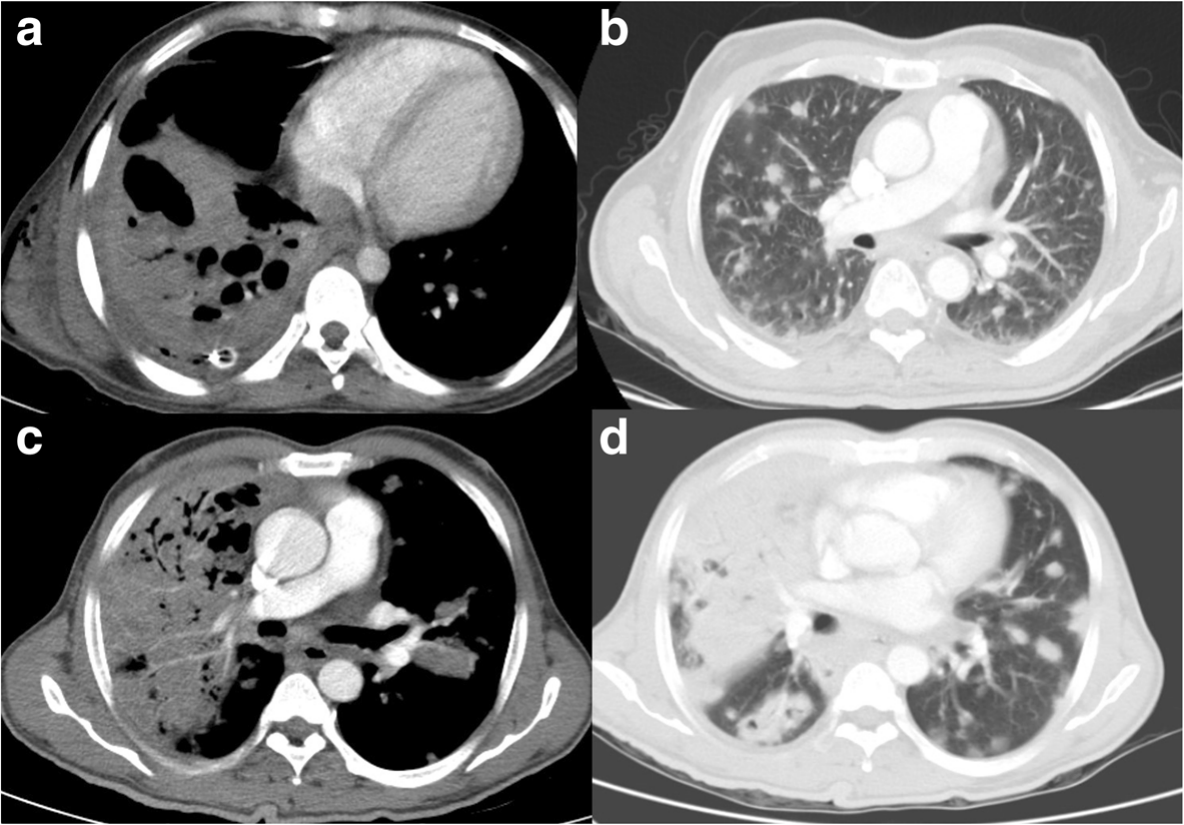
Lung melioidosis. a Forty-three-year-old male with a 1-month history of fever, respiratory symptoms, (a) axial CT thorax showed large consolidation with cavitation, intercostal drainage tube with residual mild right sided effusion, small chest wall collection. b Sixty-five-year-old male, known diabetic, presented with fever and low oxygen saturation at the time of admission, axial CT performed depicts multiple nodules in the lungs. This patient succumbed to death on day 6 of admission. Axial CT performed for a 49-year-old male presented with a 7-day history of fever, sputum culture grew Burkholderia pseudomallei, blood culture grew *Pseudomonas aeruginosa*, CT thorax in (c) mediastinal and (d) lung windows show large, multisegmental consolidation with cavitations and multiple nodules. Despite starting treatment for both organisms and being on ventilator support, he died on day 5 of admission
